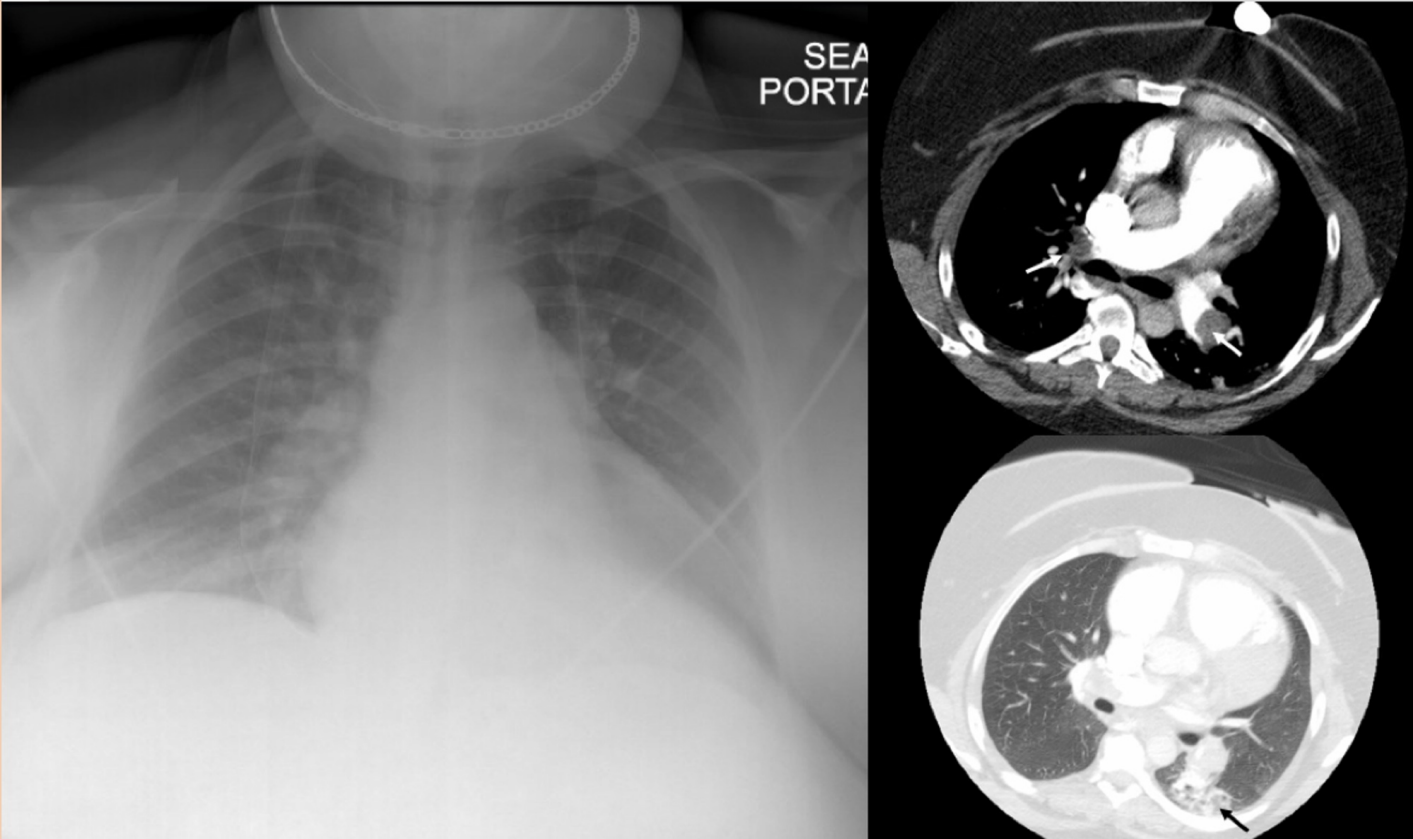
CXR revealed no significant infiltration (left), CT chest angiogram revealed thrombus in bilateral pulmonary arteries (white arrow) and peripheral ground-glass appearance (black arrow)
